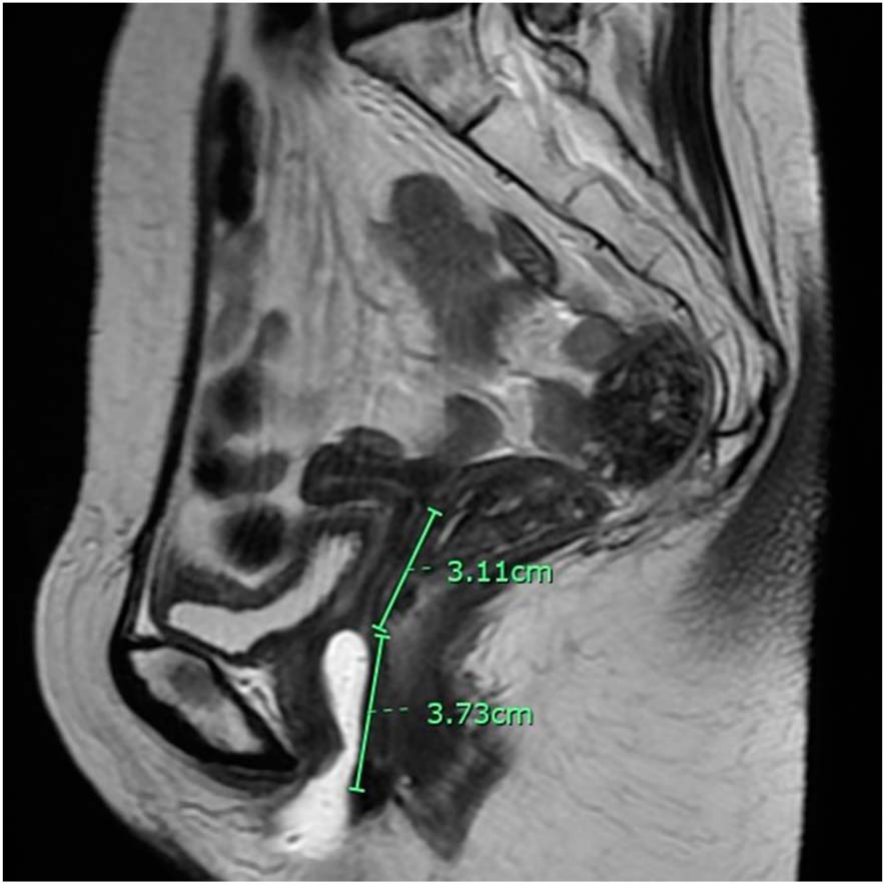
Sagittal T2-weighted magnetic resonance image showing 3.7-cm vagina length and 3.1-cm closed vaginal apex.
